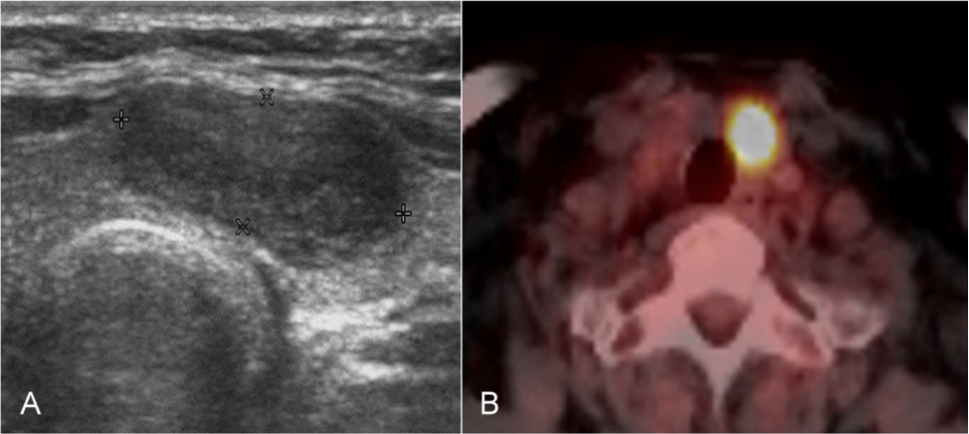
Images of follicular carcinoma. a On ultrasonography, there is a 1.7-cm, hypoechoic nodule. b Focal, intense ^18^F-FDG uptake in the left isthmic nodule on ^18^F-FDG PET/CT (SUVmax = 11.97)
